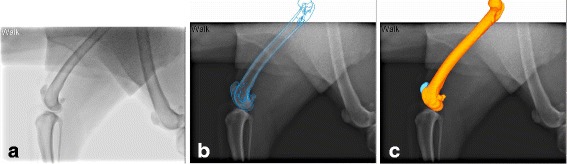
Image registration process. a Flat-panel radiographic image imported into JointTrack^e^. b Contoured silhouettes based on the 3-D models of the femur and patella utilized for shape-matching over the radiographic image. c Shape-matched 3-D projections of the femur and patella
